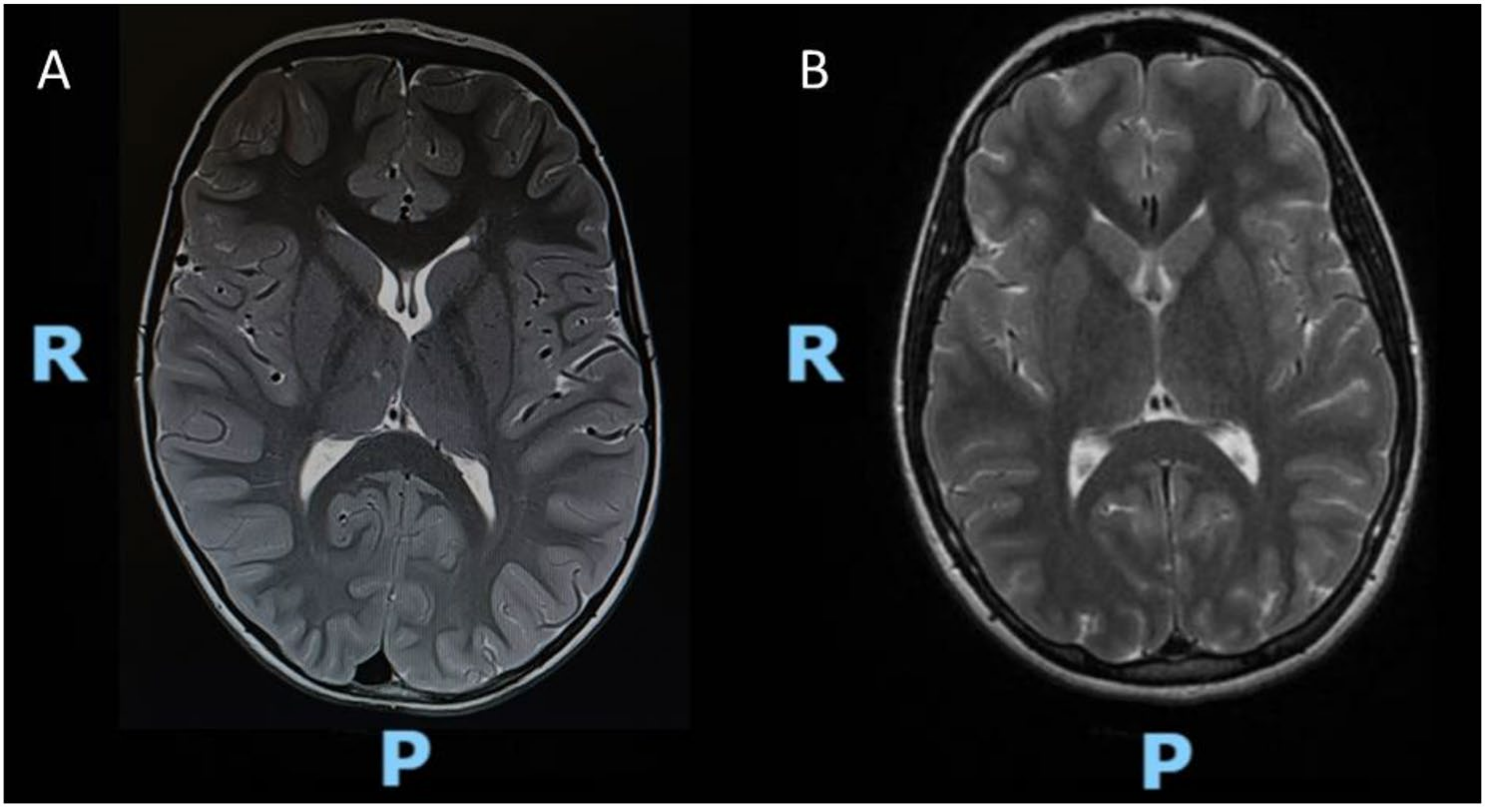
A-B. Brain MRI of the present patient at the onset during status epilepticus and at the age of 11 y.o. (T2-weighted images). A. The initial MRI at five years old showed mild hyperintensity in peri-trigonal white matter on T2-weighted sequences. B. This finding subsequently resolved on the follow-up MRI at eleven years old. Legend: R=right side; P= posterior side
